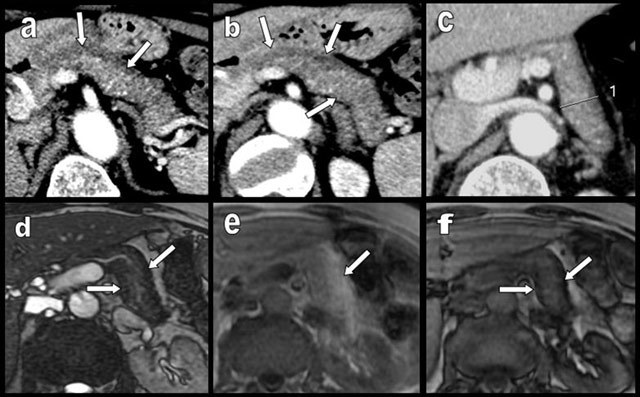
Atypical peripheral uneven FI. The gland (a and b) is surrounded by an hypodense band mimicking peripancreatic edema or a subtle effusion on contrast enhanced CT views (white arrows on a and b). This hypodense band was absent 2 years before, and the pancreatic tail was thinner (c). The peripheral band is hypointense on a T2-weighted image (d), thus excluding edematous effusion (white arrows on d). Axial in-phase images (e) show normal signal intensity in the body of the pancreas, higher signal in the peripheral gland, markedly on the anterior side (white arrow on e), with a drop of signal intensity on opposed-phase imaging (white arrows on f) revealing atypical peripheral uneven pericaudal FI.
